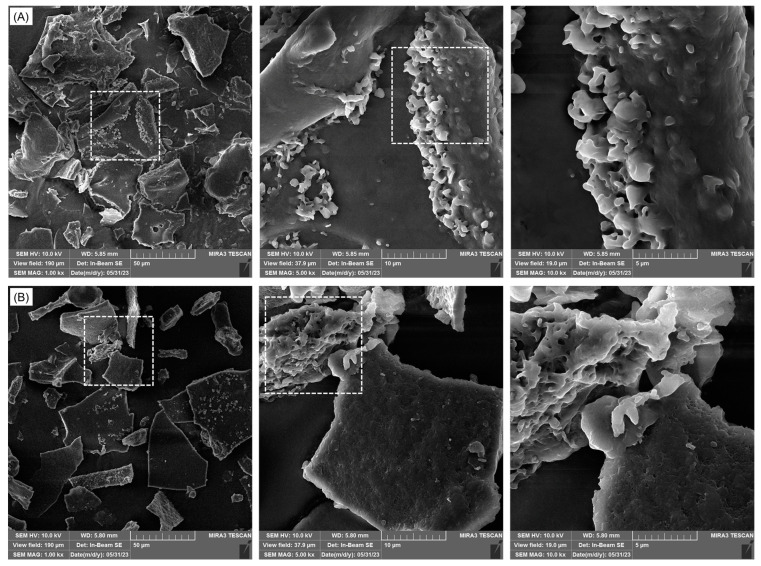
FESEM images (magnification 1000, 5000, and 10,000× from left to right) of RuBisCO-rich proteins extracted from pumpkin leaves by maceration (A) and high-intensity ultrasound with an amplitude of 40% (B).
